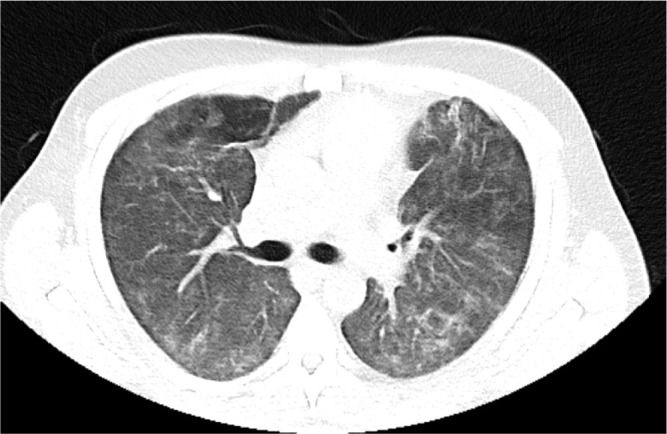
CT Thorax performed Day 92 prior to discharge home showing further improvement of both ground glass opacification and reticular shadowing with minor traction bronchiectasis.
